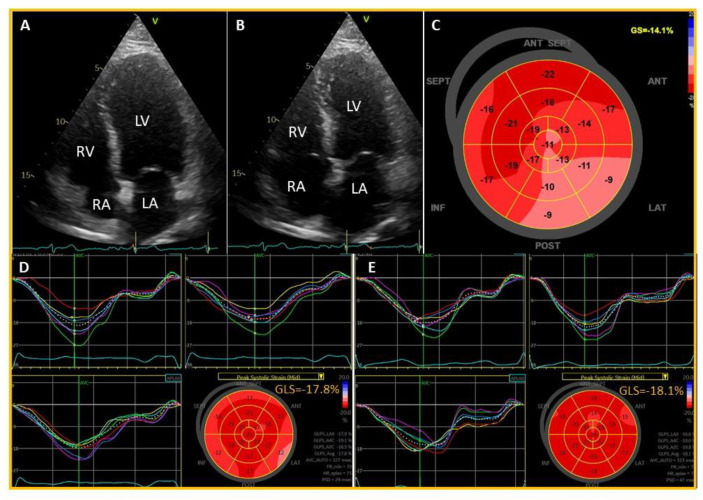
Echocardiographic images of the heart on admission (A–C), at discharge (D) and at 6-weeks follow-up (E). (A) demonstrates end-diastolic and (B) end-systolic frames on apical four chamber views. The distal septal segments towards the apex are hypokinetic and slightly dilated (A) and shows little motion toward the mid-cavity during end-systole (B). The Bulls eye plot shows reduced (less negative) strain values in lateral and posterior walls from basal to apical segments (C) with an average global longitudinal strain (GLS) of −14.1%. (D) displays improvement of GLS to −17.8% on discharge but still some impaired strain value in a basal segment of the lateral wall. Strain values were further improved to −18.1% at 6-weeks follow-up (E), although there was some post-systolic shortening (left upper and lower panels). LA, left atrium; LV, left ventricle; RA, right atrium; RV, right ventricle.
